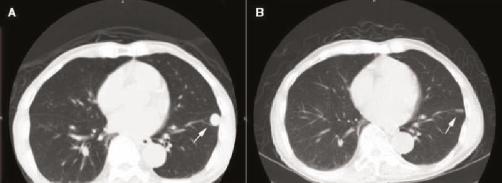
(A) The pulmonary metastasis in the left lower zone of the lung 6 years post-nephrectomy. (B) A CT scan one year post relapse showing near complete regression of the lesion.
